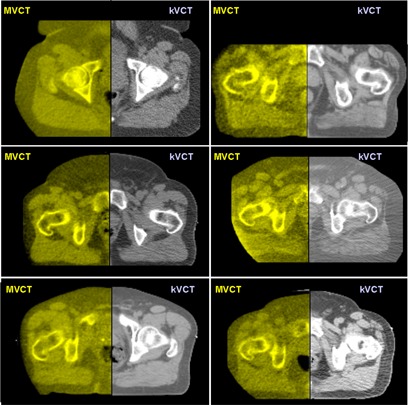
Six random examples of axial megavoltage computed tomography (MVCT)–to–kilovoltage computed tomography (kVCT) registration for prostate patients using the “checkerboard” image fusion tool on the TomoTherapy (Madison, WI) operator station. The right half of each prostate image is the reference kVCT (grayscale), and the left half is the daily MVCT image (yellowscale). Note that the prostatic–rectal interface is visible in each image.
